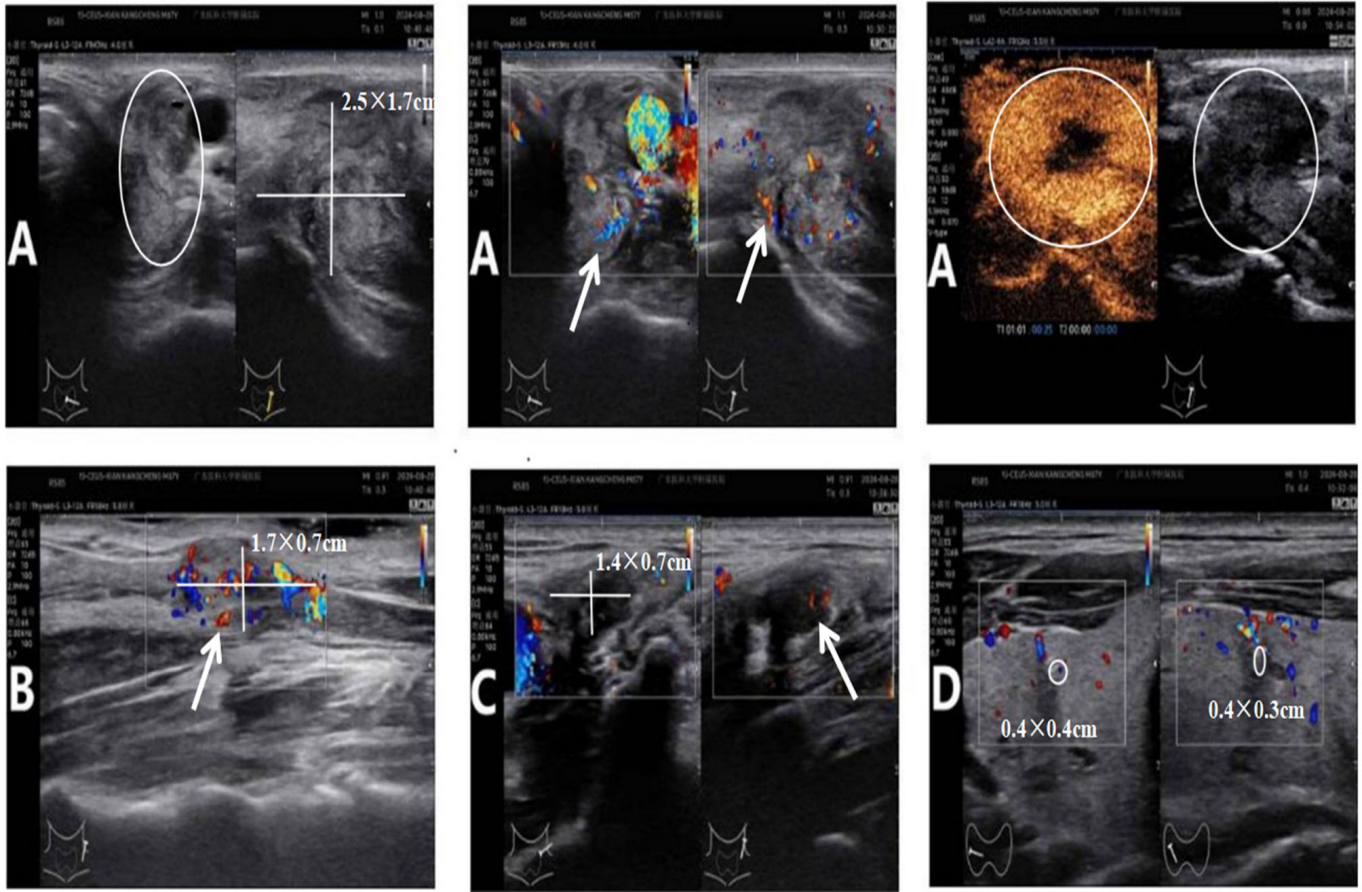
Preoperative contrast-enhanced cervical ultrasound images. Symbol key: white arrows = blood-flow signals on Doppler/CEUS; white circles/ellipses = outlined lesion; white crosshair calipers = linear measurements shown on the panel. (A) Two orthogonal views of a hypoechoic lesion in the left thyroid bed; the lesion is outlined by a white ellipse, and white crosshair calipers show 2.5 × 1.7 cm (third dimension 2.2 cm by orthogonal view); color Doppler views of the same lesion, with white arrows indicating blood-flow signals around/within it; contrast-enhanced images with the lesion circled in white. (B) A hypoechoic lesion located superolateral to the left common carotid artery; white arrows mark blood-flow signals, and white crosshair calipers show 1.7 × 0.7 cm. (C) Multiple hypoechoic lesions in left level II with partial fusion; white crosshair calipers show 1.4 × 0.7 cm for the index cluster, and a white arrow marks representative blood-flow signals. (D) Small solid hypoechoic lesion(s) in the right thyroid lobe (white circles), with the displayed sizes 0.4 × 0.3 cm and 0.4 × 0.4 cm.
